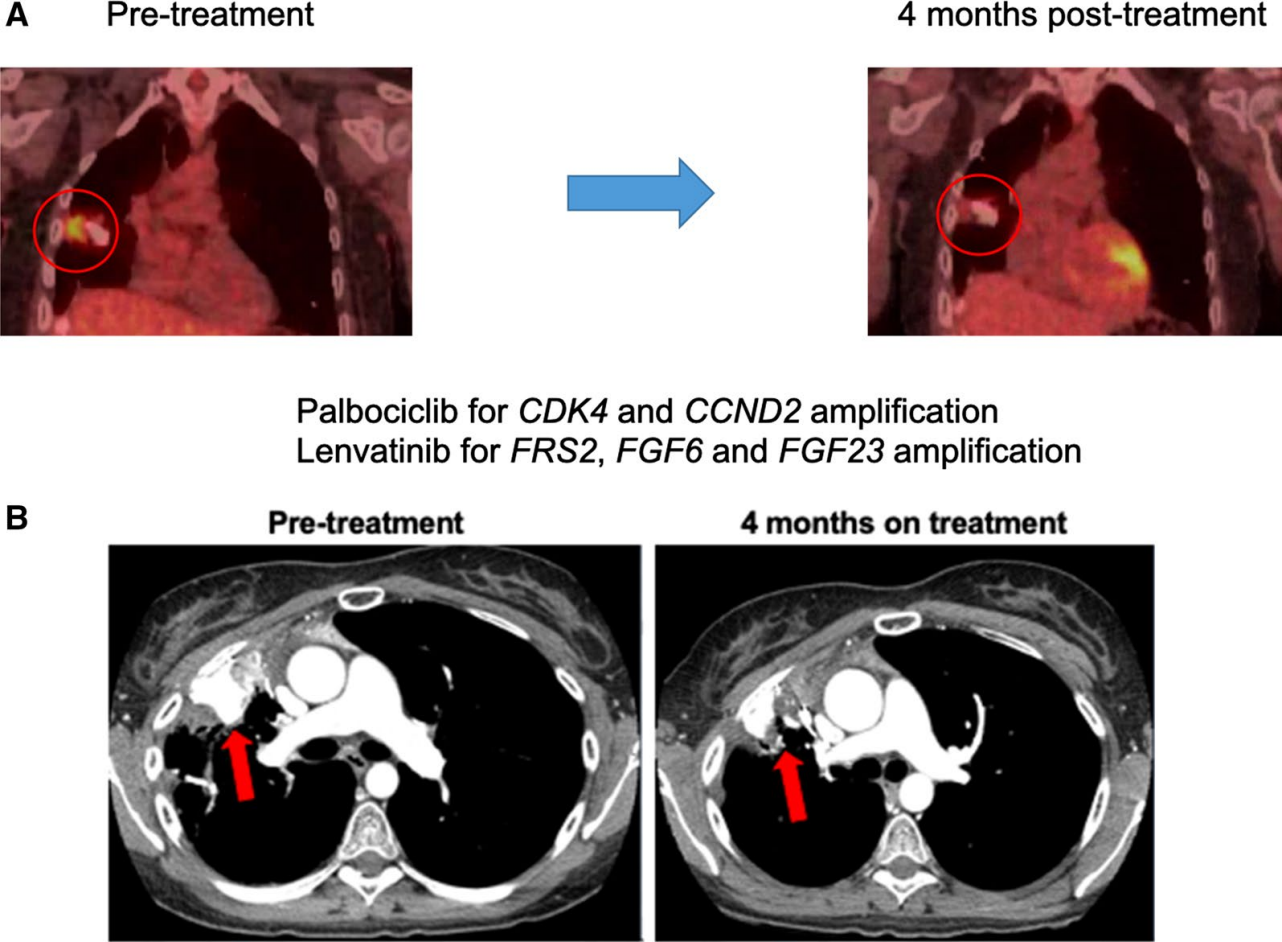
Osteosarcoma patient with multiple recurrences whose tumor progressed on several lines of therapy, now treated successfully with matched targeted combination treatment approach. Therapy ongoing at 51 + months. A (PET scan) and B (CT scan). Osteosarcoma patient with multiple recurrences whose tumor progressed on several lines of therapy, now treated successfully with matched targeted combination treatment approach. Therapy ongoing at 51 + months
